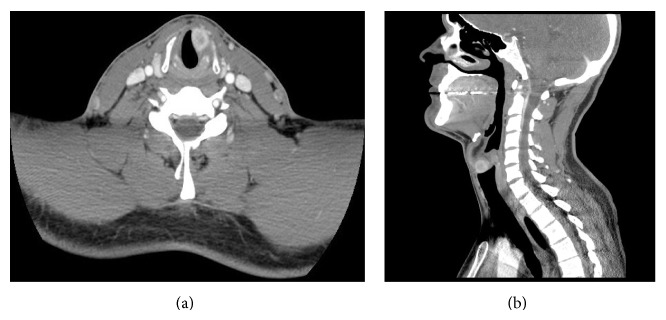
Axial (a) and sagittal (b) CT of the avidly enhancing mass in the anterior left vocal cord.
